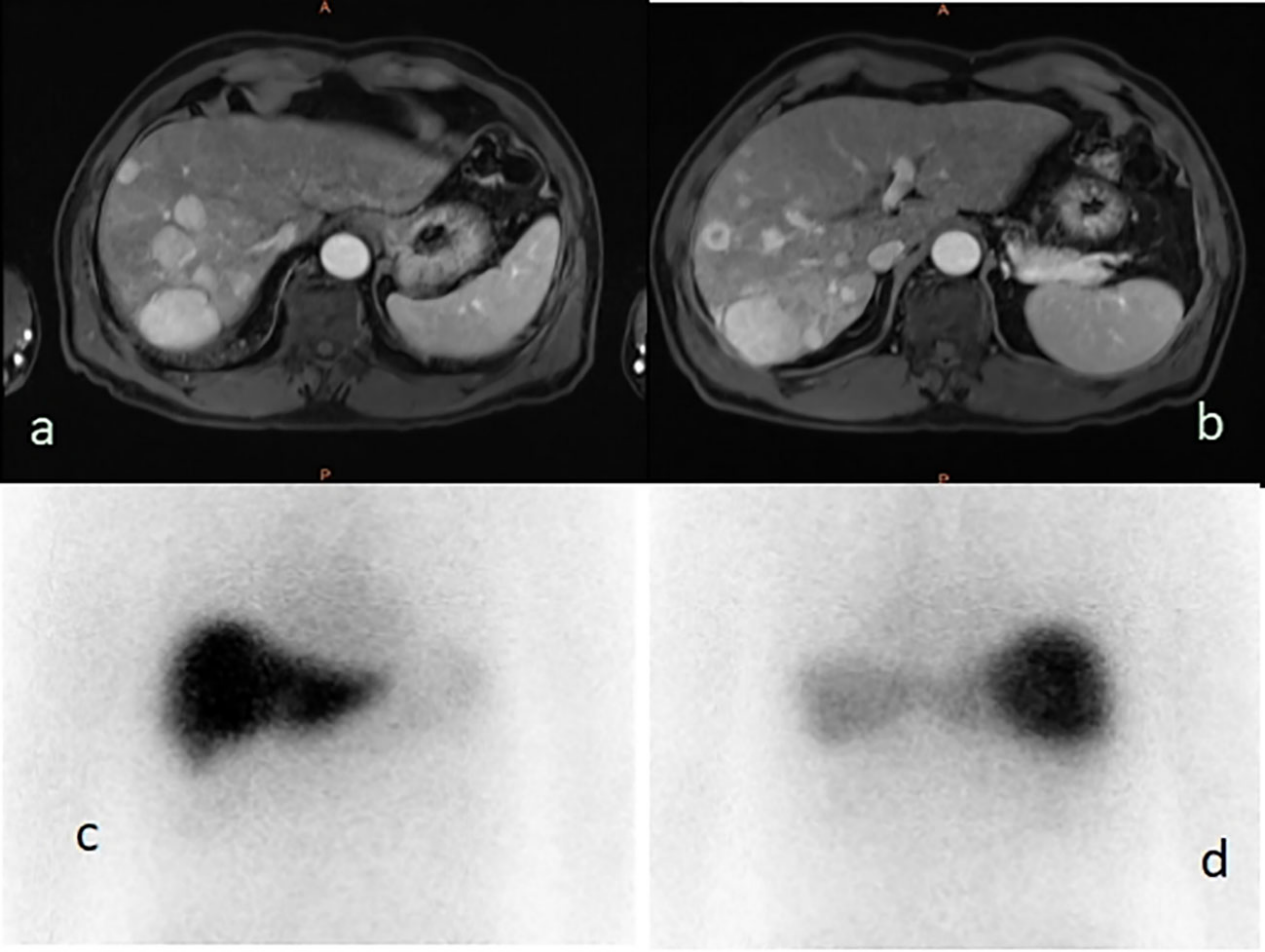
(A, B) The liver magnetic resonance imaging demonstrated multiple parenchymal nodules in right lobe of liver (5.0 × 3.8 cm^2^); (C) The 131-I whole body scan showed that the right and left lobes of the liver were evenly uptake of 131-I before, and the multiple nodules showed no higher uptake than the surrounding liver tissues; (D) The 131-I whole body scan showed that the right lobe of the liver was evenly uptake of 131-I, and the multiple nodules showed no higher uptake than the surrounding liver tissues.
